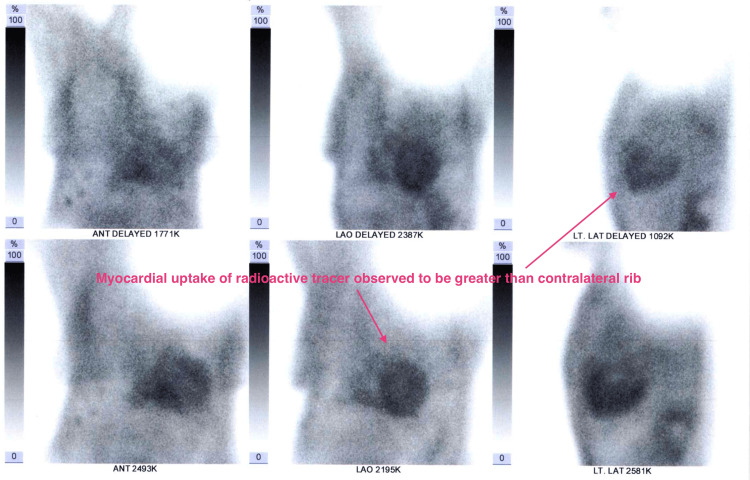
Cardiac scintigraphy showing increased myocardial uptake of the radioactive tracer ANT: anterior, LAO: left anterior oblique, LT LAT: left lateral
